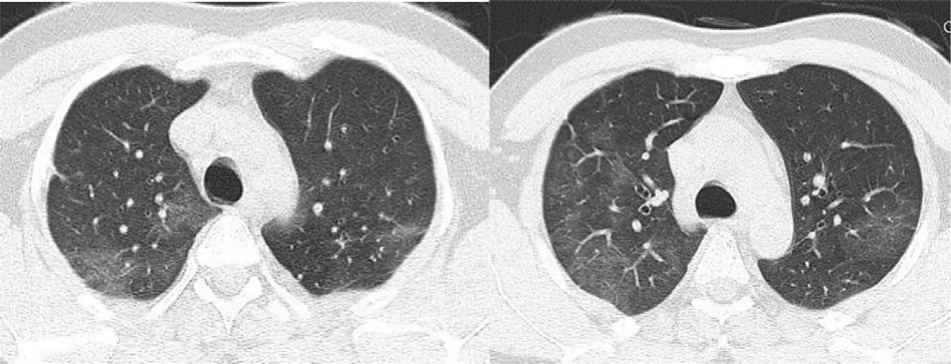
Chest CT scan on 30th day of illness.
